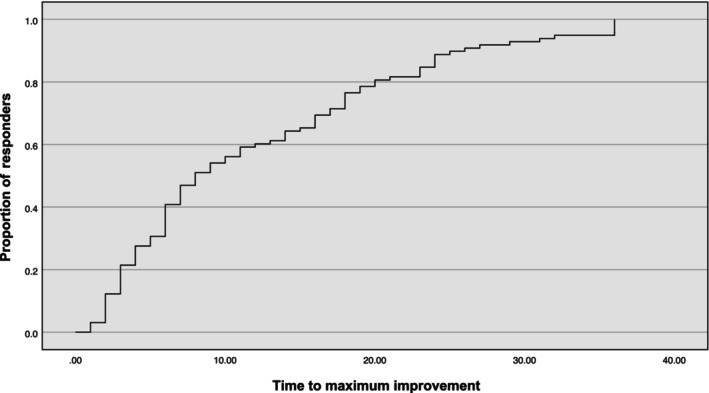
Proportion of subjects achieving maximum response within a 36‐month follow‐up period in 112 consecutive subjects with chronic inflammatory demyelinating polyneuropathy (CIDP) receiving routine care. CT‐RC, CIDP treatment‐response category; MID, minimum clinically important difference; ONLS, Overall Neuropathy Limitation Score.
